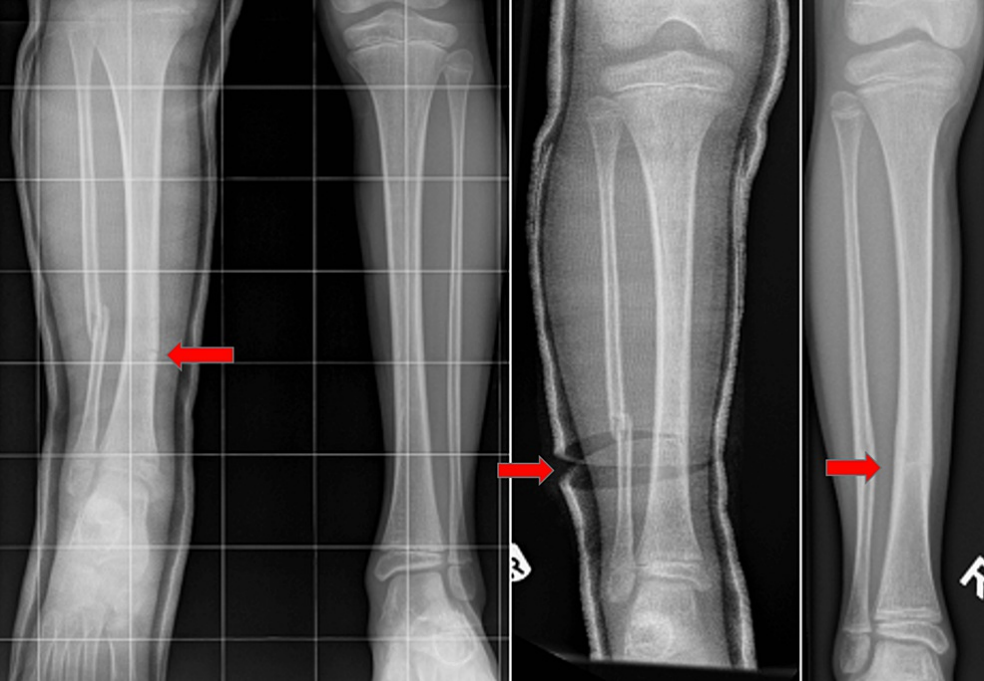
An 11-year-old boy with fracture of tibia diaphysis and fibula at the same level. From left to right: fracture angulated at two weeks’ review following MUA in theatre (radiographs in left), wedge created in the casts (radiograph in the middle), and satisfactory healing and alignment four months after initial casting (radiograph in the right). Arrow in the first radiograph shows angulation at the fracture site in the anteroposterior view. Arrow in the third picture shows wedging of the cast resulting in correction of angulation. Arrow in the fourth picture shows satisfactory healing with acceptable angulation at the fracture site. MUA, manipulation under anaesthesia
